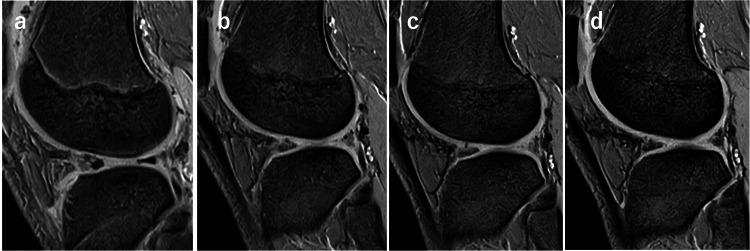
The change over time of the tendon graft on the sagittal view of MRI (a) Post-operative at 10 days; (b) post-operative at three months; (c) post-operative at six months; (d) post-operative at two years.
